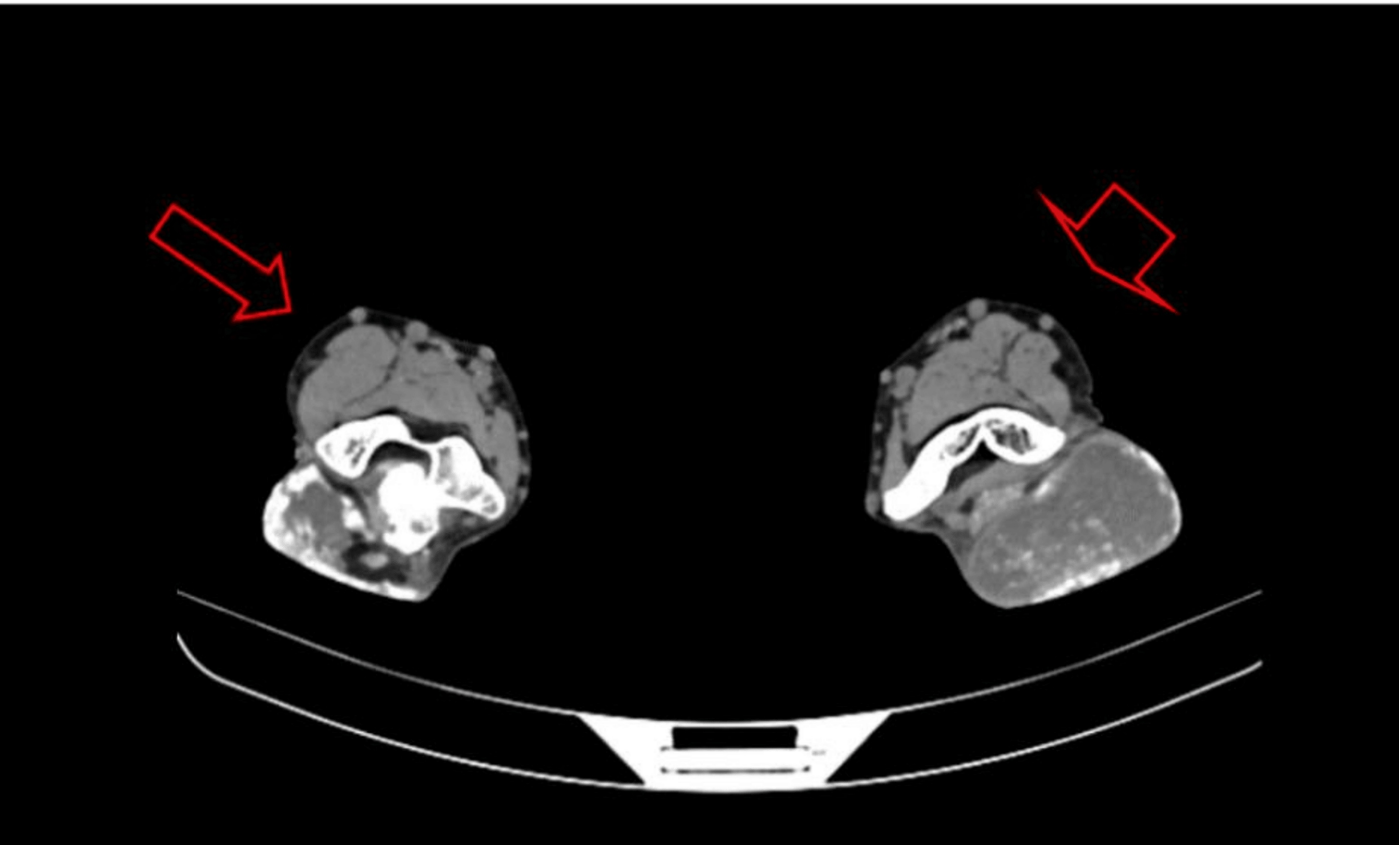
CT scan axial view of both elbows showing bilateral soft tissue fluid-filled cystic masses
